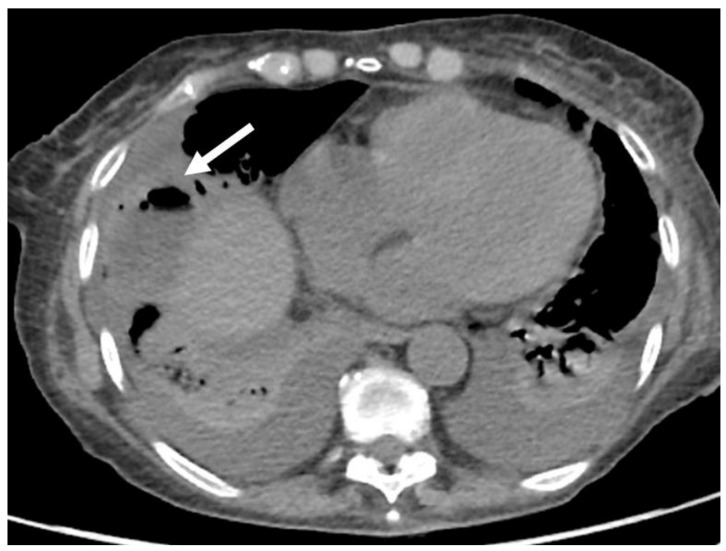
CT assessment at 1-week follow-up after radio frequency of HCC located on segment VIII. The arrow shows pulmonary abscess in patient with diaphragm damage.
